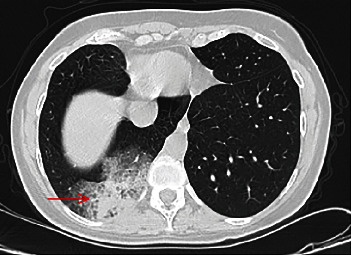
Axial view of the lower chest CT showing the consolidation and the ground glass opacity in the posterior segment of the right lower lung representing the sequestered lobe (red arrow).
